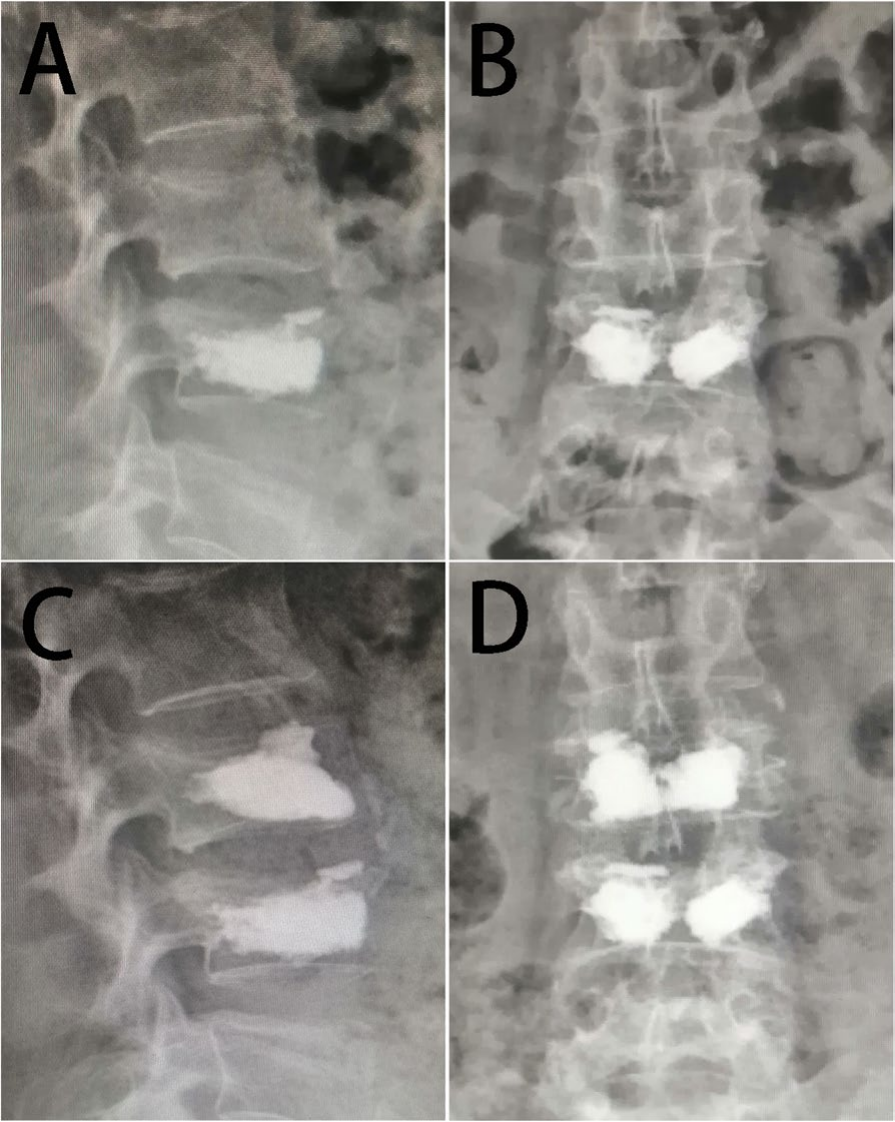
A and (B) are the lateral and anteroposterior X-rays after the first fracture operation; (C) and (D) are the lateral and positive X-rays after the second fracture
